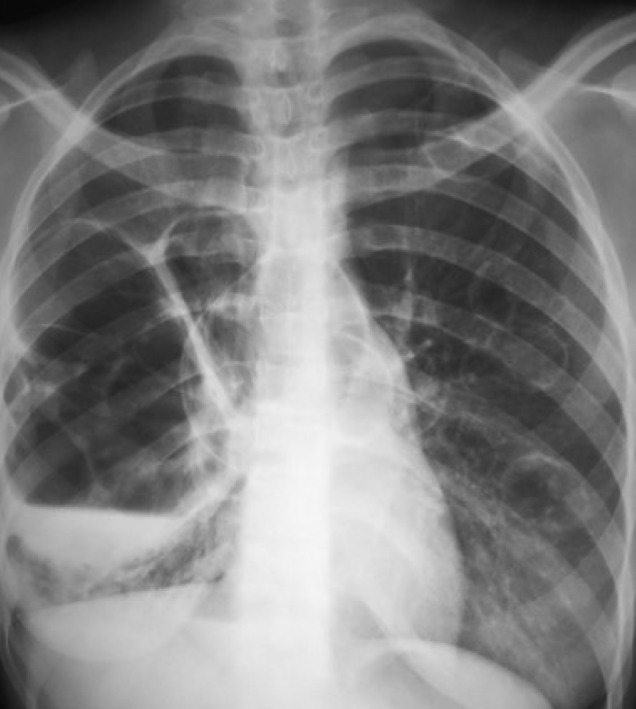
Chest X-ray showing multiple cysts.
